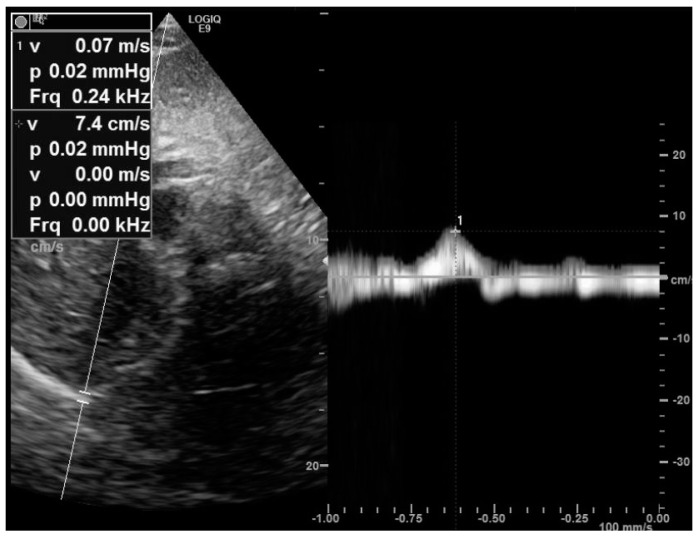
Measurement of the right peak sniff tissue Doppler imaging velocity from the subcostal view. The diaphragm velocity was recorded during a sniff maneuver. Here is a reduced peak sniff velocity (7 cm/s) in a patient with muscular dystrophy.
